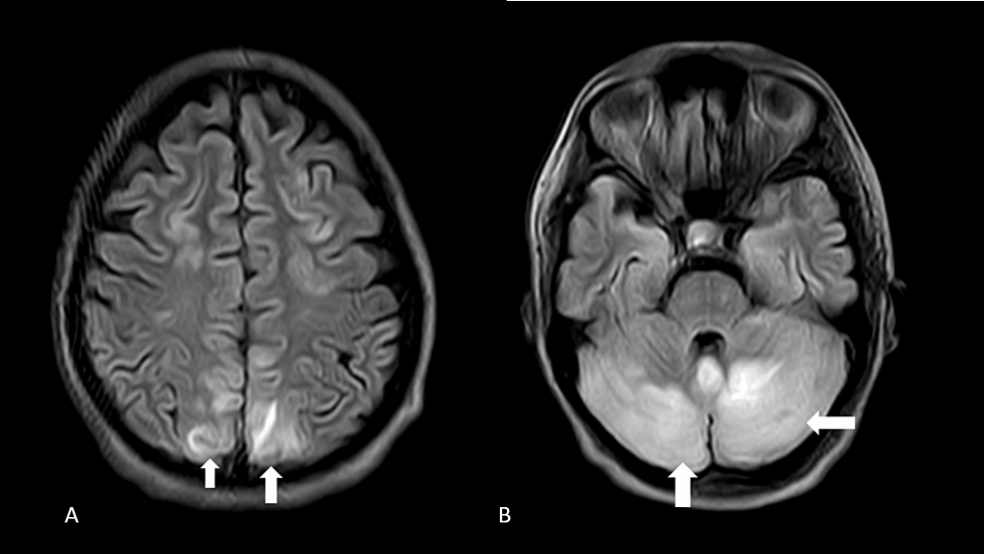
MRI brain; T2 FLAIR sequence showing cortical white matter signal hyperintensity foci (white arrows) in bilateral occipital, parietal regions (A) and bilateral cerebellar hemispheres (B). FLAIR: Fluid attenuated inversion recovery
